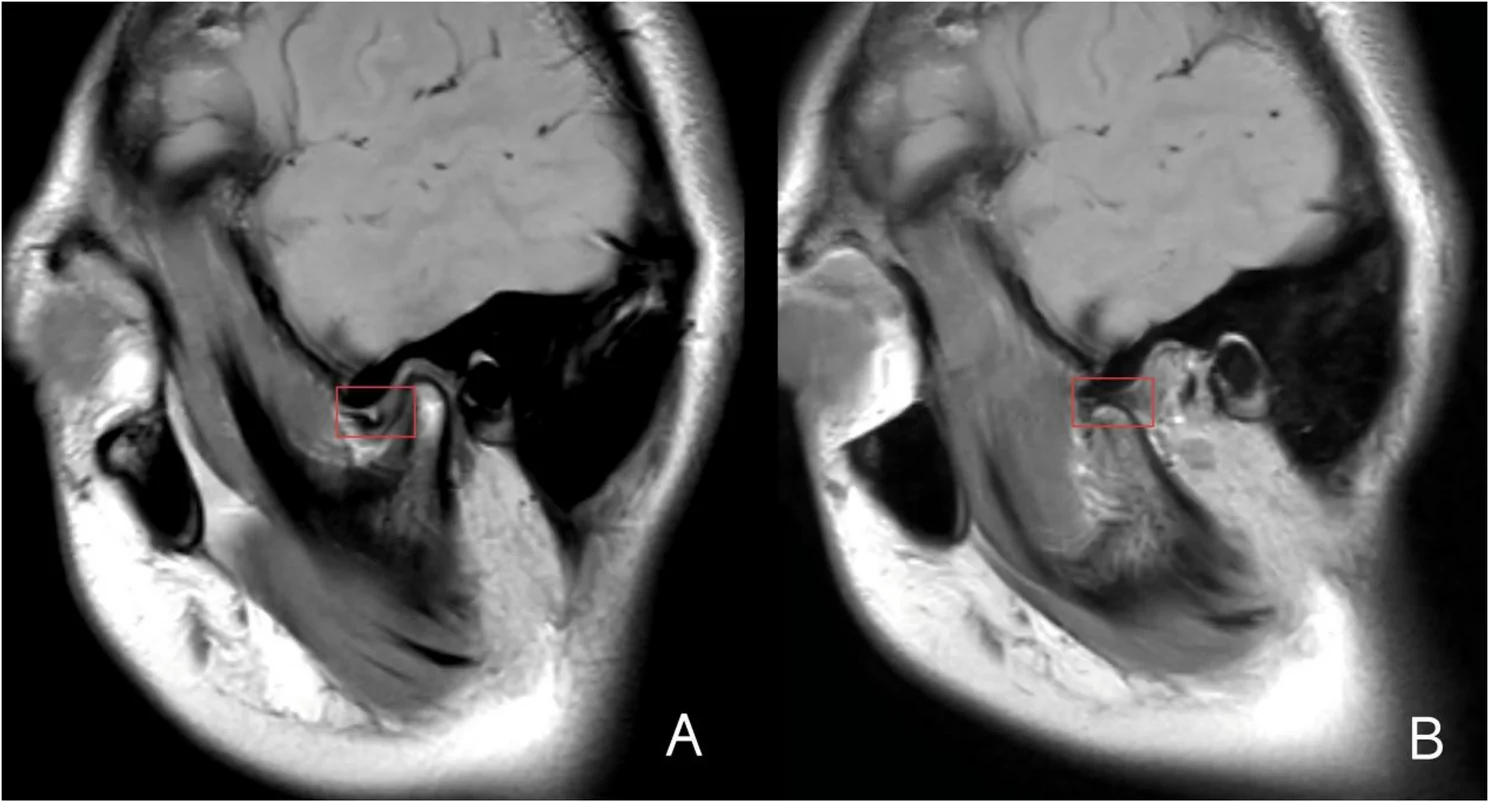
Representative MRI of an ADDwR patient: (A) closed-mouth position showing anterior disc displacement (red box); (B) open-mouth position showing disc reduction
